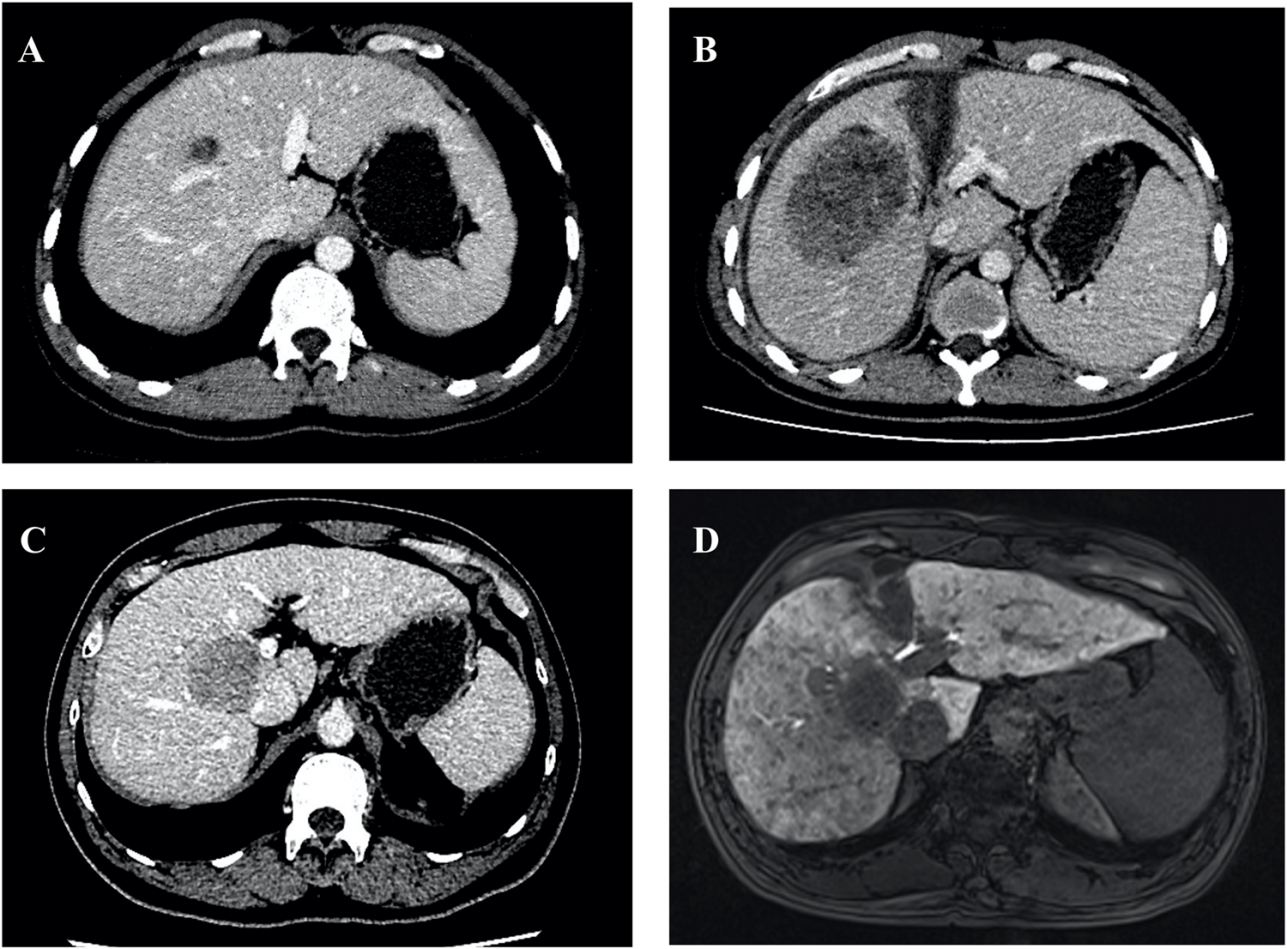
Liver regeneration negatively correlated with fibrosis/cirrhosis. A A 32-year-old male patient with mild fibrosis and his preoperative CT image. B The same patient in A with mild fibrosis. The CT taken 7 days after ALPPS Stage 1 showed that the volume of the left lobe is 49.47% of the standard liver volume, with a kinetic growth rate (KGR) of 46.84 mL/d. C A 48-year-old male patient with cirrhosis and his preoperative CT image. D The same patient in C with cirrhosis. The hepatobiliary phase of MRI in 78 days after ALPPS Stage 1 showed that the volume of the left lobe is 35.84% of the standard liver volume, with a kinetic growth rate (KGR) of 7.61 mL/d, which is significantly slower than that of the patient with mild fibrosis
